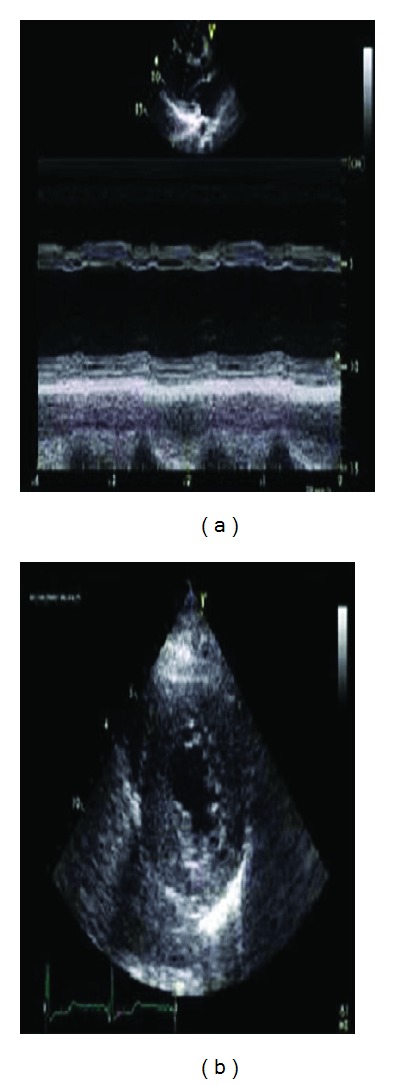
(a) Parasternal long-axis view showing the left ventricular dilatation and advanced global hypokinesis. (b) Parasternal short axis view showing the septal and posterior wall hypertrophy.
